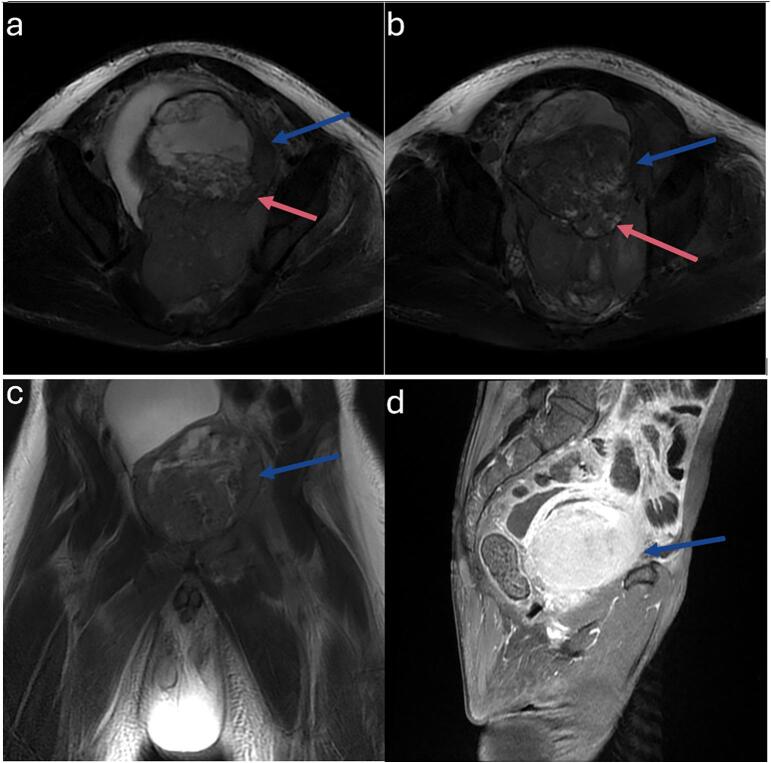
a & b) Axial T2; c) Coronal T2; d) Sagittal T1: MRI scan showed an irregular parietal thickening of the bladder in isosignal T2, T1 and hypersignal on diffusion sequences, clearly enhanced by contrast medium, developed at the expense of the bladder trigone and the posterior wall of the bladder and budded endoluminally creating a mass filling almost the entire bladder lumen. It measures 81 ∗ 78 ∗ 105 mm (blue arrow). The process protruded beyond the posterior bladder wall, breaching the latter and invading the left seminal vesicle (red arrow).
